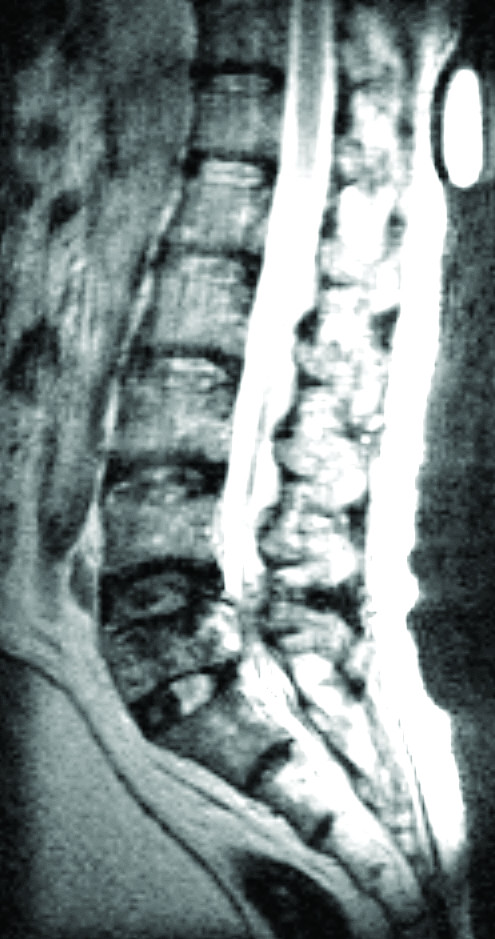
Magnetic resonance image of lumbosacral spine showing a compression fracture of L5 of posterior bulging contour with enhancement and epidural soft tissue mass over the anterior epidural space with marked compression of the thecal sac.
